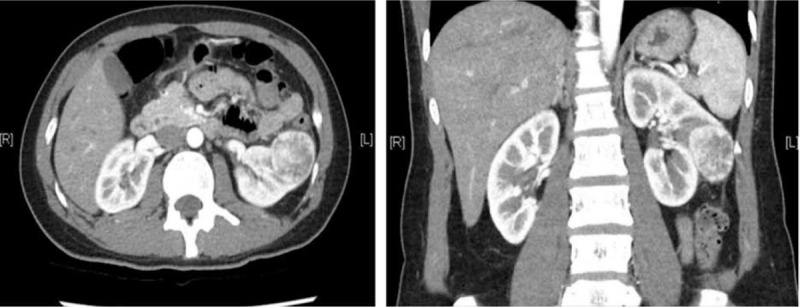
Preoperative CT scans showing the renal tumor to be treated with zero ischemia robot-assisted partial nephrectomy: A 51-year-old female patient with a left 3.6 cm exophytic midpole renal tumor.
